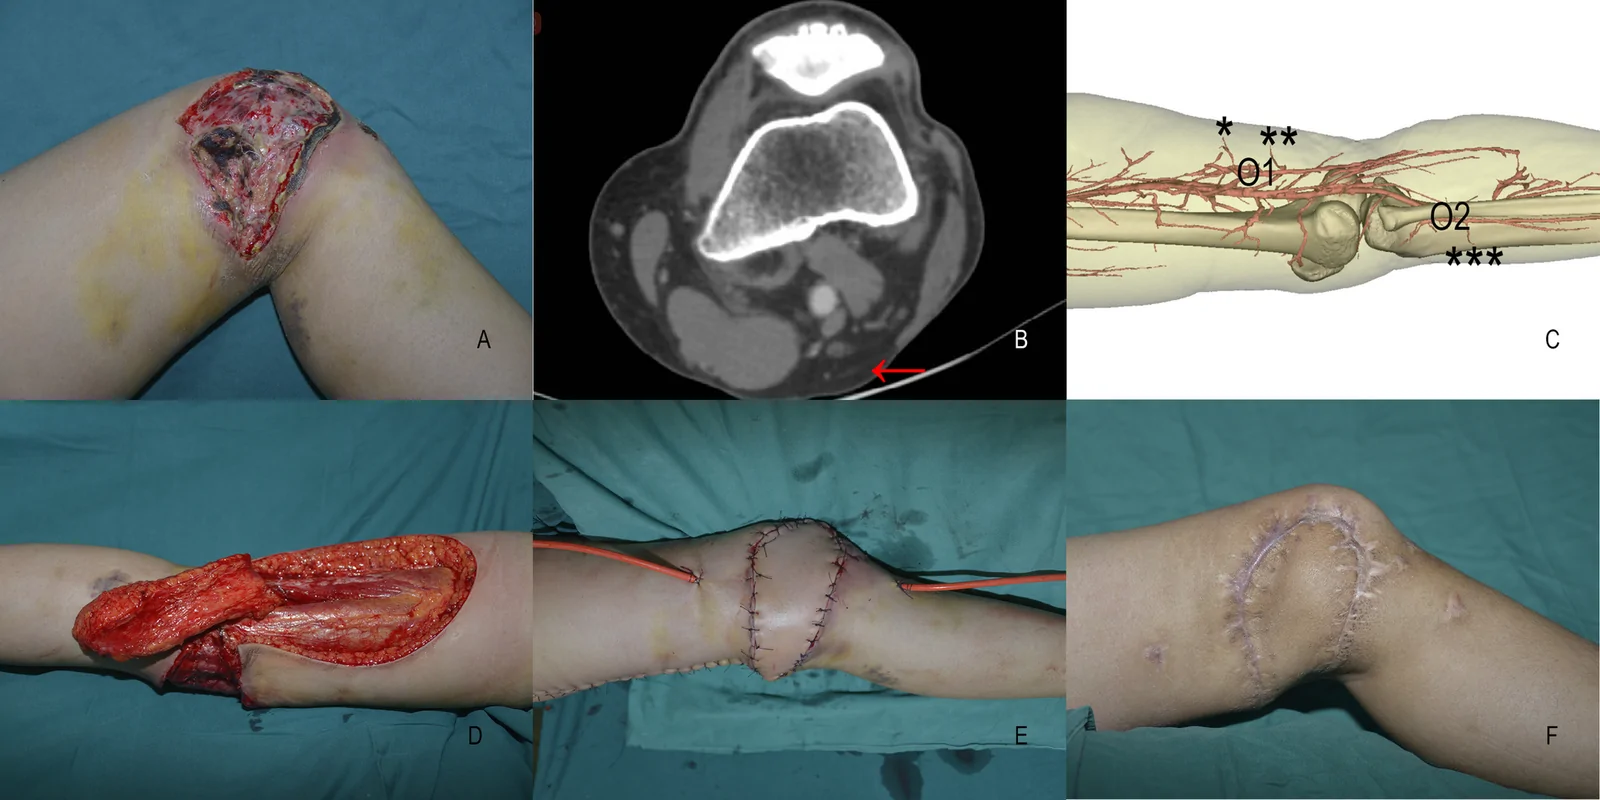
(A) Preoperative view of the soft tissue defect on the medial aspect of the left knee. B Preoperative CTA shows the location of perforator 2, indicated by the red arrow; this intermuscular perforator measured 2.1 cm in length. C Mimics 20.0 reconstruction showed two peri-popliteal perforators, one of which was interpreted as arising from the popliteal artery according to CTA-visible source-vessel continuity, and one anterior tibial artery perforator. O1: popliteal artery; O2: anterior tibial artery; *: perforator 1; : perforator 2; *: perforator 3. D An 18 × 7 cm peri-popliteal perforator flap was harvested without muscle separation. E Immediate postoperative appearance. F Photograph of the affected limb 1 year postoperatively
